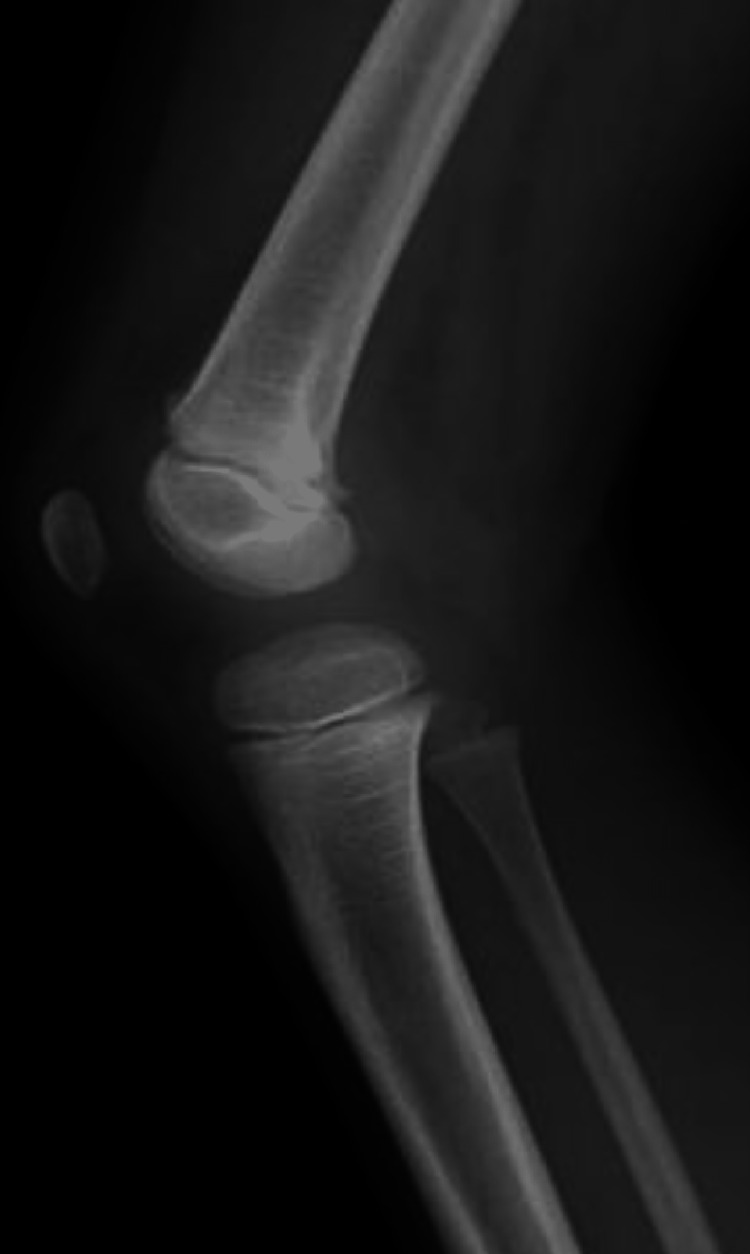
A six-year-old boy with staphylococcal osteomyelitis The lateral plain radiograph shows no obviously abnormal findings [17].
